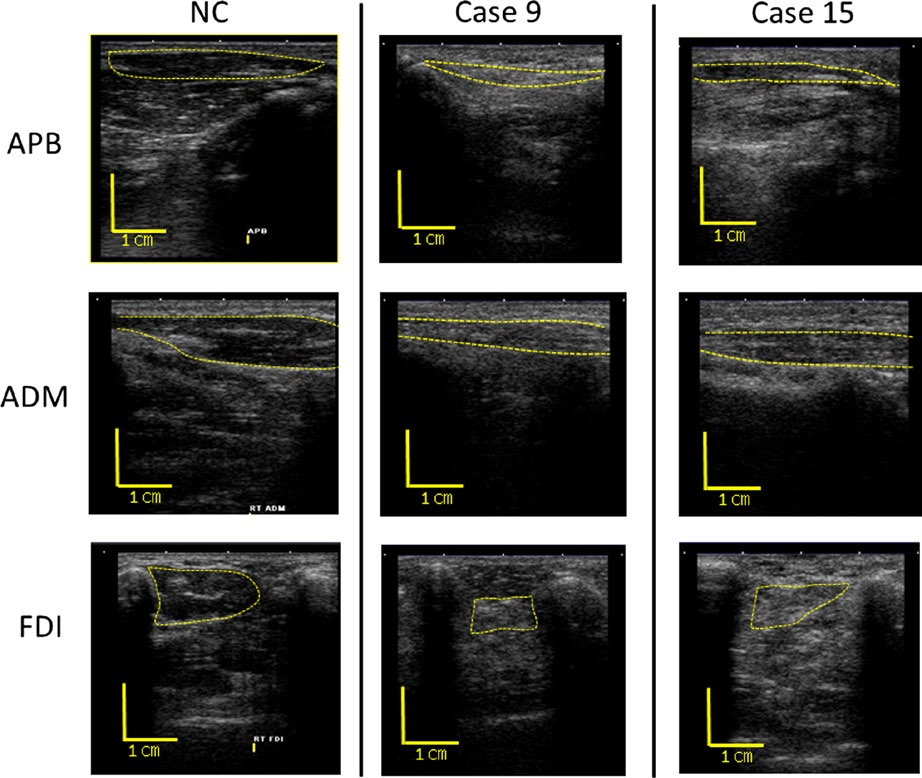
Visual MUS findings. NC: Typical views and findings of each muscle in an NC. Each muscle was surrounded by a broken line. The parenchyma of every muscle appeared black, indicating low echo intensity. Cases 9 and 15: The muscle parenchyma of each muscle appeared white (indicating high echo intensity), and the size of muscles was smaller than that in an NC. MUS, muscle ultrasound; APB, abductor pollicis brevis; FDI, first dorsal interosseous; NC, normal control
